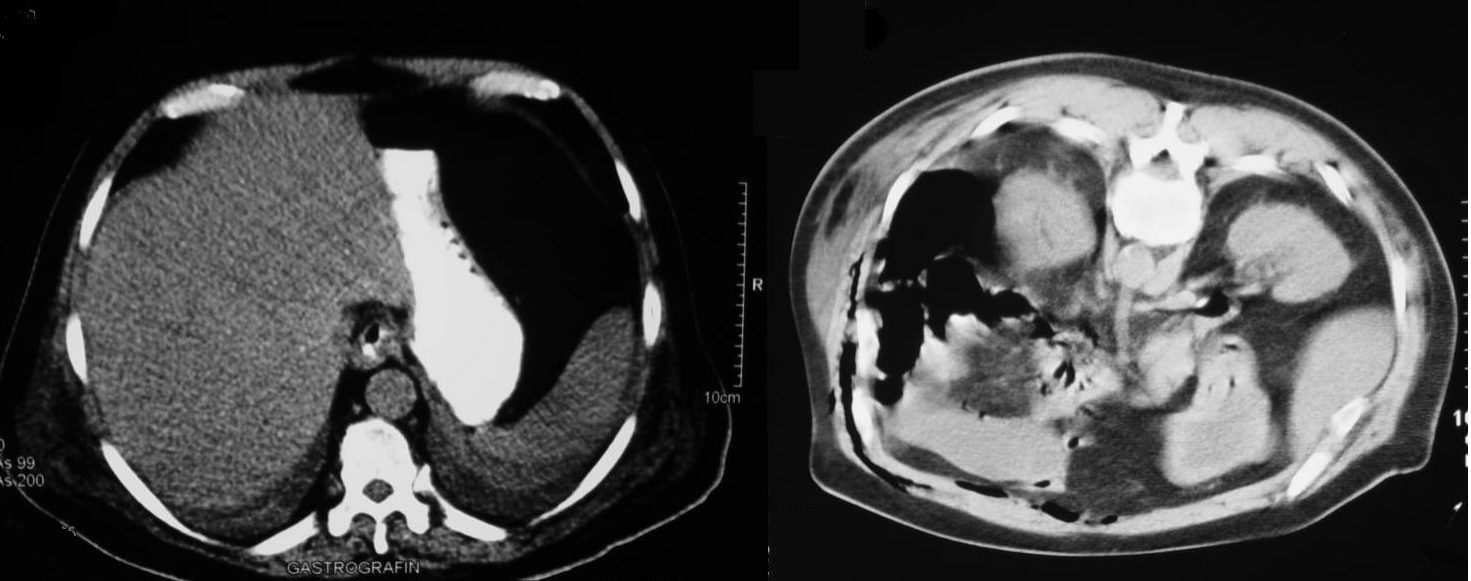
Abdominal CT; intraperitoneal air in the upper abdomen sets the diagnosis of acute abdomen necessitating surgical exploration.
